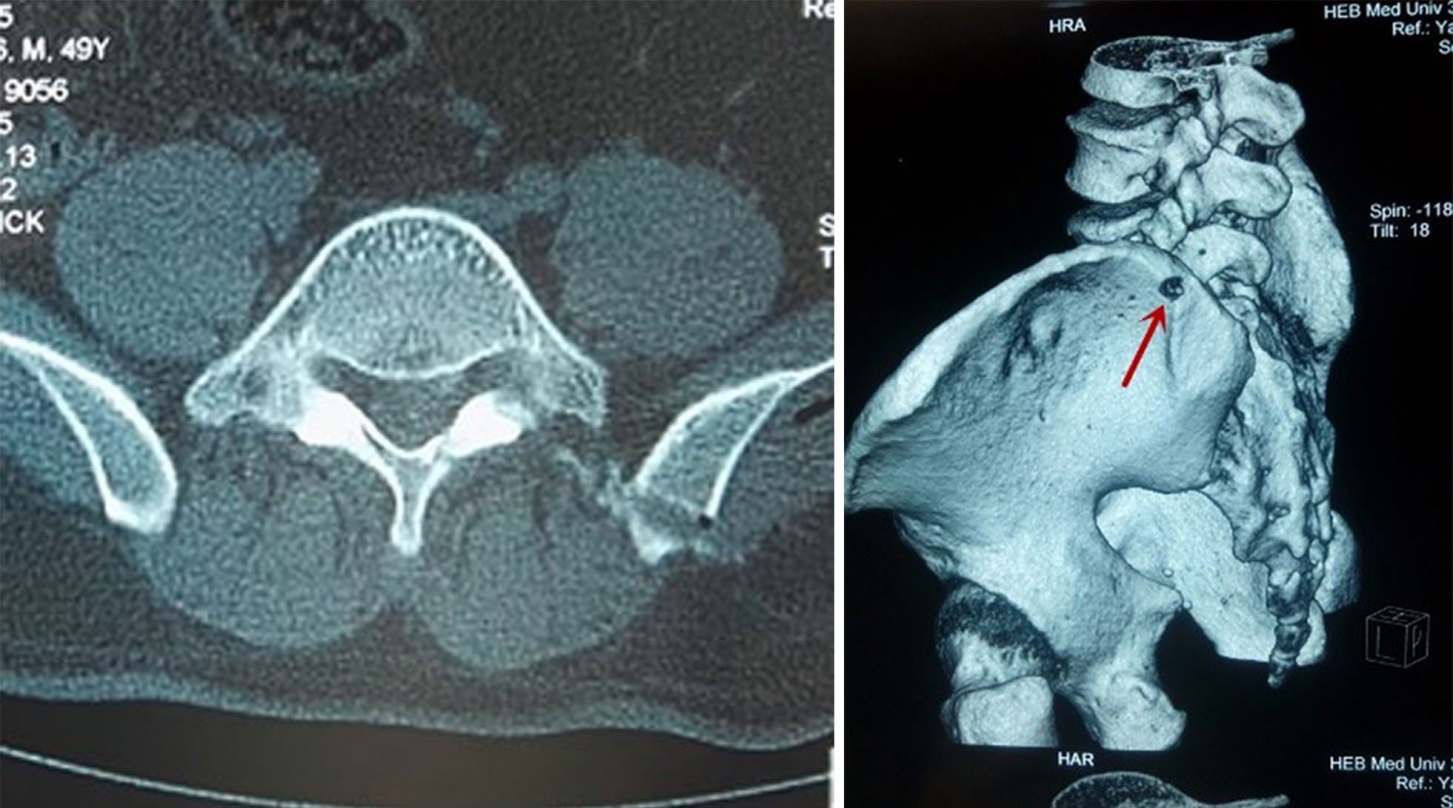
The CT ilium channel postoperatively. *Left* The vertebral CT scans after transiliac approach to intervertebral endoscopic discectomy and the bone channel was seen clearly. *Right* The vertebral CT three-dimensional reconstruction after transiliac approach to intervertebral endoscopic discectomy. Where the *arrow* is located is the bone channel
